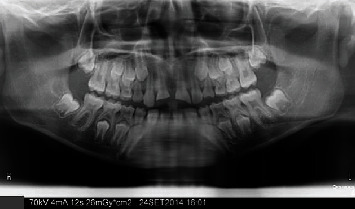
Panoramic radiography 3 days after trauma.
